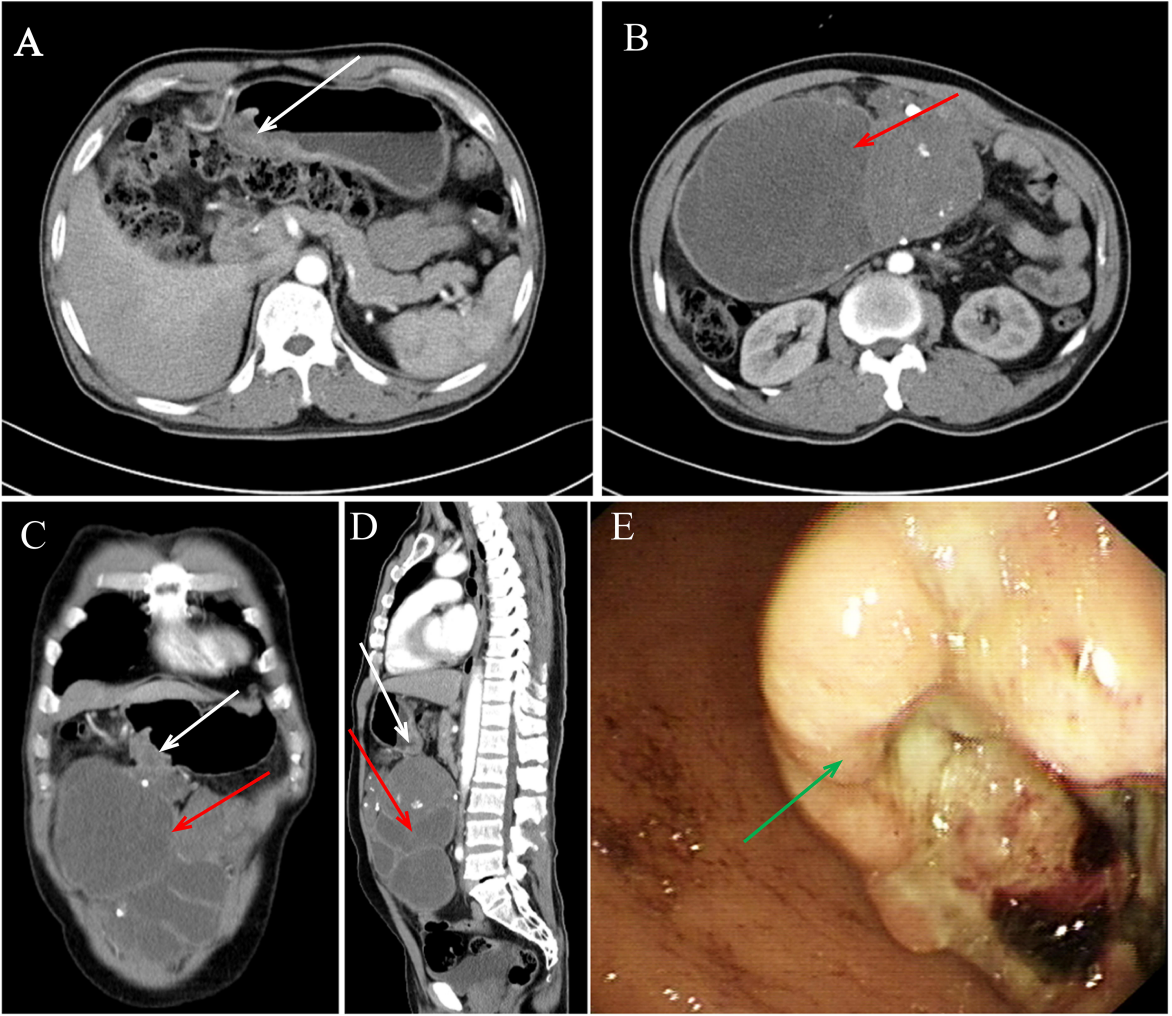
Imaging and gastroscopy findings. (A–D) Abdominal CT showed gastric wall thickening (white arrows) and a mixed solid-cystic mass (red arrows) along the greater curvature, with mild enhancement on contrast-enhanced imaging. (E) Gastroscopy confirmed a tumor at the gastric antrum-body junction (green arrow).
